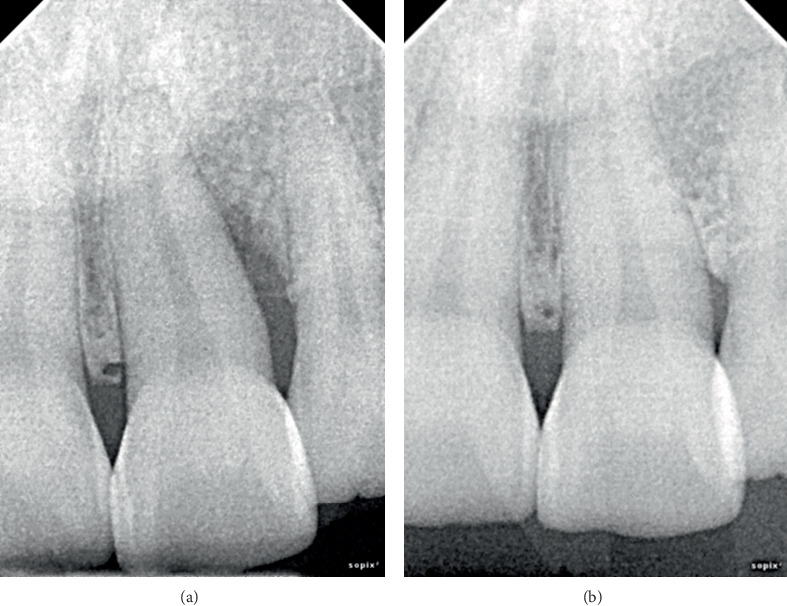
Clinical presentation of Case 6. (a) Preoperative radiograph showing an intraosseous defect on the distal side of Tooth #21. (b) Radiographic at 8 years postsurgery showing the intrabony defect filled with hard tissue.
